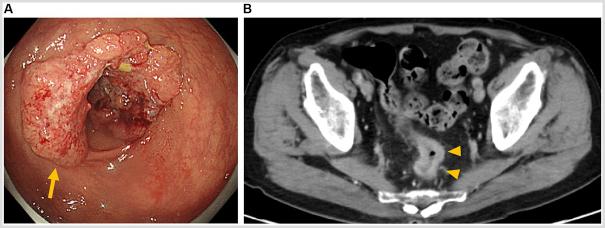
Preoperative findings. (A) Colonoscopy findings. A type 2 lesion is observed in the rectum situated 13 cm from the anal verge (arrow). (B) Abdominal contrast-enhanced computed tomography image depicting thickening of the intestinal wall with contrast enhancement in upper rectum (arrowheads).
